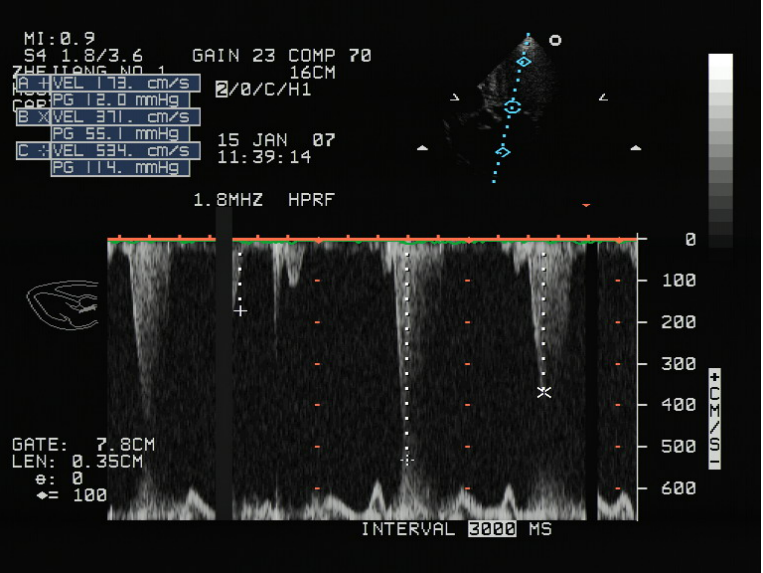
Left ventricular apical five-chamber view of showing that a dynamic left ventricular outflow tract obstruction was present, with a resting maximal instantaneous gradient of 55 mmHg (B: peak velocity, 3.7 m/s). With the Valsalva maneuver, the gradient increased to 114 mmHg (C: peak velocity, 5.3 m/s).
